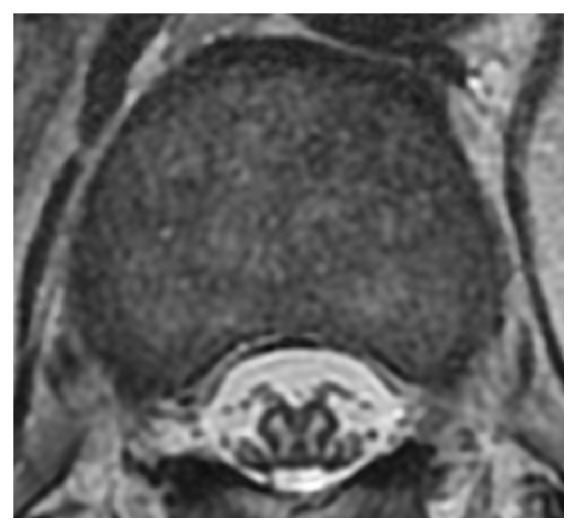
T2-weighted MRI at the T12 level showed bilateral hyperintensities in the anterior horns of the grey matter. MRI, magnetic resonance imaging.
